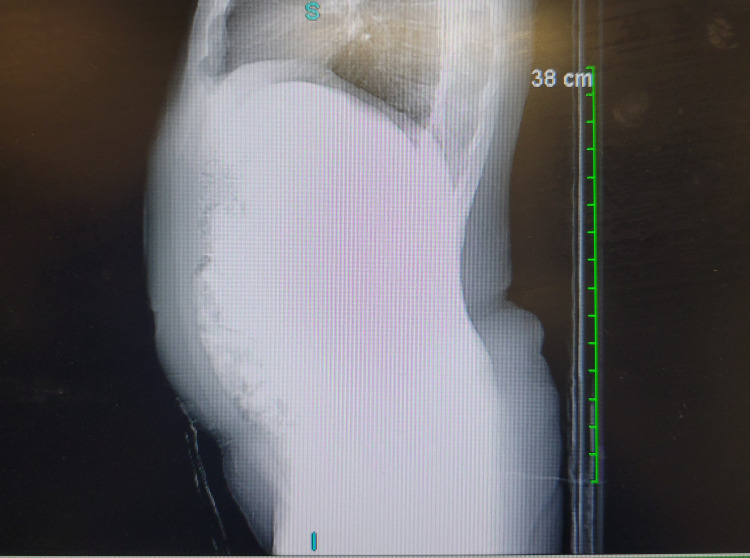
Sagittal CT image of the upper abdomen (non-contrast) obtained on June 27, 2025, demonstrating an intact fascial repair without evidence of recurrent hernia, seroma, abscess, or postoperative fluid collection Partially visualized bowel loops are not dilated. No acute intra-abdominal pathology is identified.
